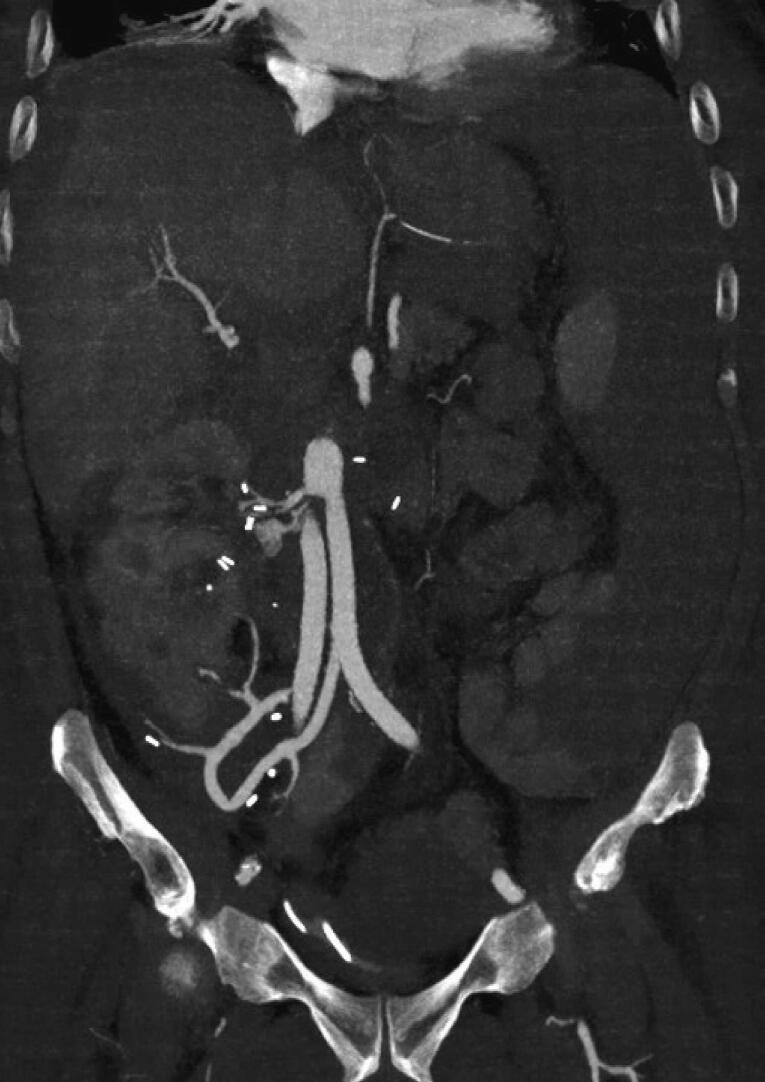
CT morphological arterial reconstruction.
